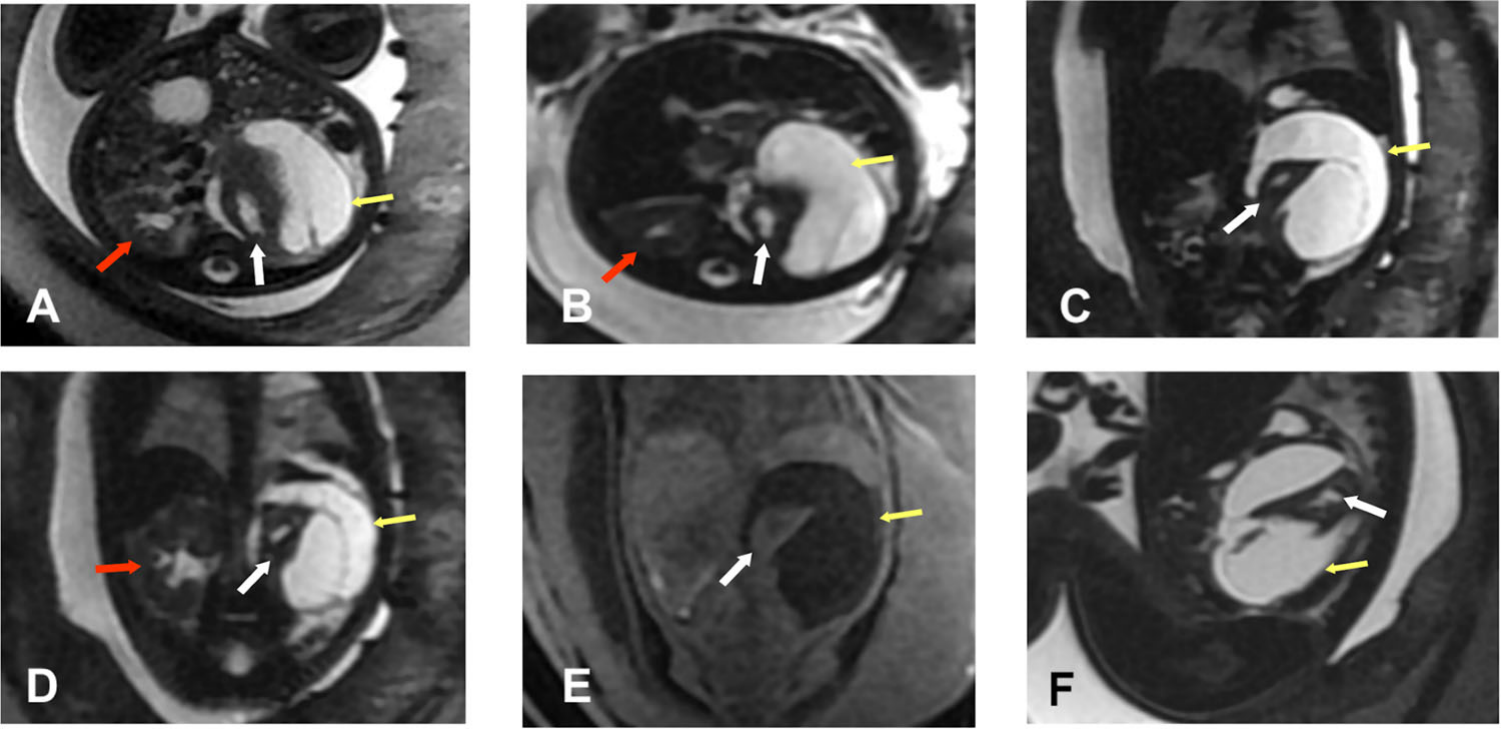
Left perirenal urinoma. Fetal ultrasound: perirenal hemorrhage or hemorrhagic cyst. A, B Axial SS FSE T2. C, D Coronal SS FSE T2. E Coronal 3D LAVA. F Sagittal SS FSE T2. Normal right kidney (red arrows). Perirenal cystic lesion (yellow arrows) surrounding the left kidney (white arrows), compressing and displacing it anteromedially. It has septations inside without signs of bleeding in the T1 sequence. There are no signs of hydronephrosis. The MRI is reported as lymphangioma or retroperitoneal teratoma. The lesion progressively disappeared after birth and corresponded to a urinoma
